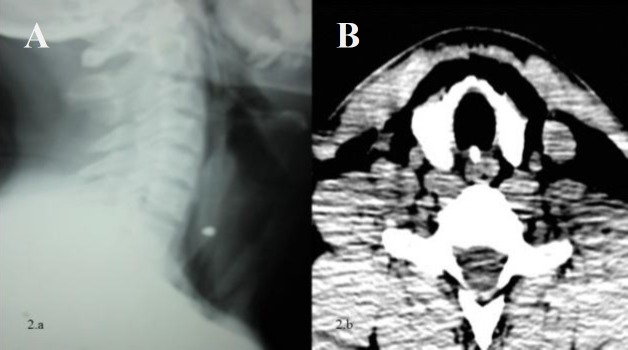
a) X-ray soft tissue neck lateral view showing the radio-opaque foreign body located in the tracheoesophageal party wall at the C6–C7 level with air lucency noted in the prevertebral space. b) CECT scan neck showing radio-dense metallic foreign body located in the trachea-esophageal party wall with air dissection in the subcutaneous plane
